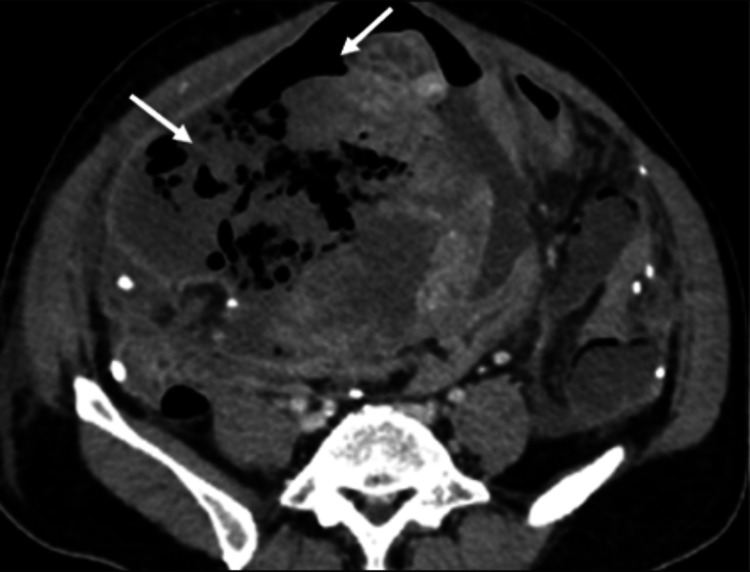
Axial CECT image in venous phase demonstrating exophytic mass with nondependent intratumoral air foci (white arrows) (Torricelli-Bernoulli sign) CECT: contrast-enhanced computed tomography
